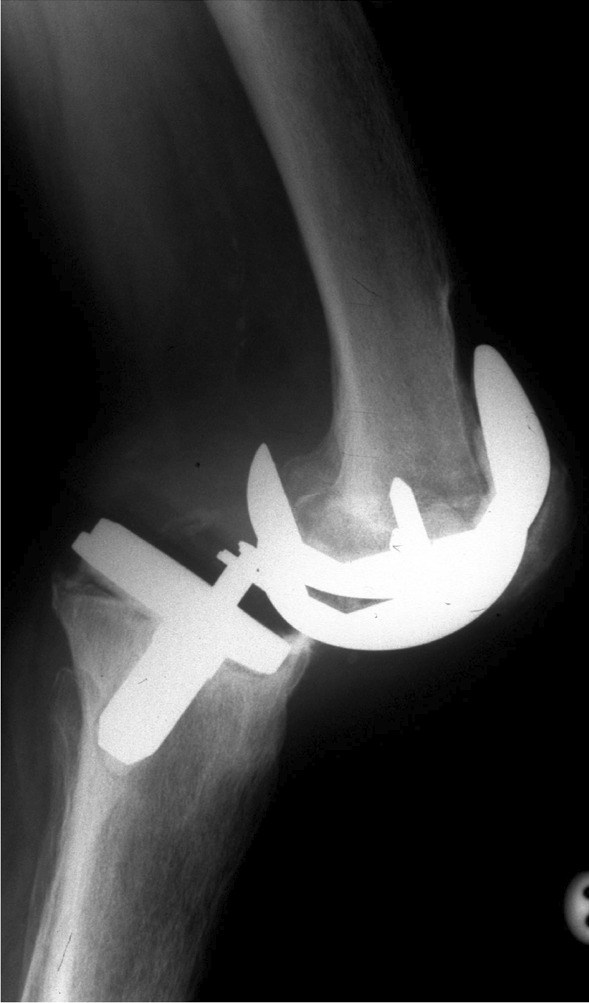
This lateral radiograph demonstrates significant loosening and collapse. The trigger for this presentation was flexion instability which progressed to this presentation. This underlines the importance of early recognition of instability and addressing the appropriate cause
